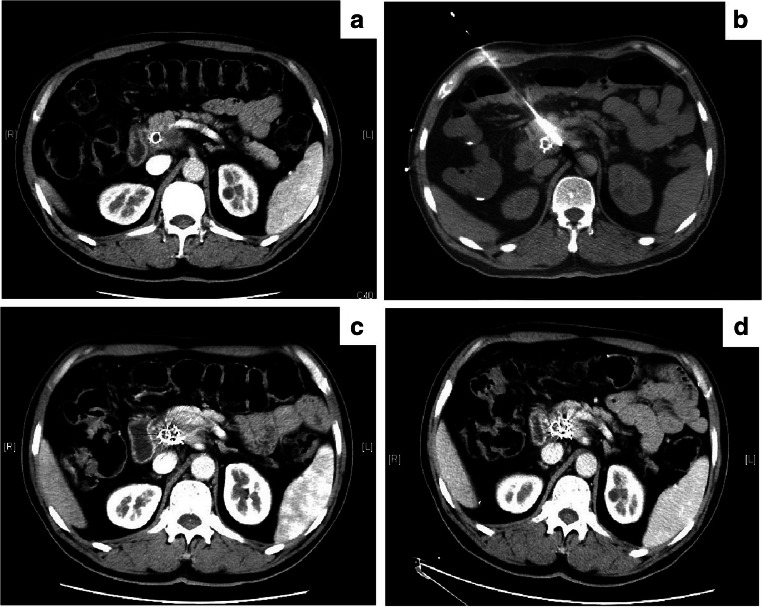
A 60-year-old male with pancreatic cancer at the neck, and the spleen artery was involved in. a Abdominal CT revealed the tumor before the treatment. b Intraoperative puncture procedure under the CT-guided. c, d The local lesion achieved complete response (CR) after 2 months (c) and 6 months (d) follow-up
